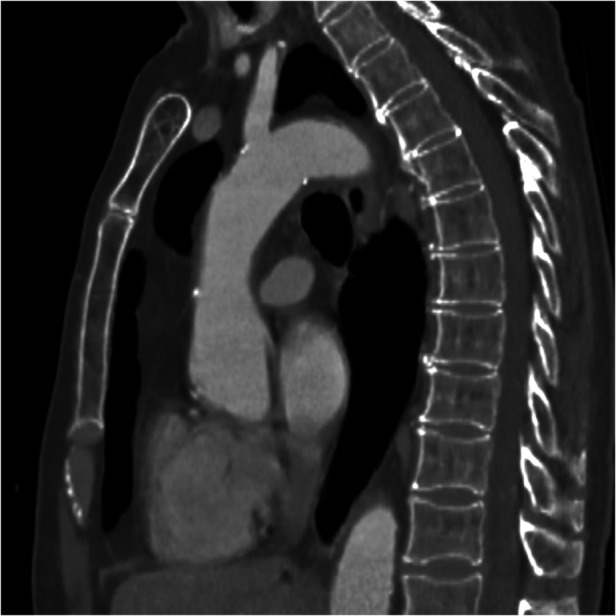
Sagittal CT-scan plane showing the resolution of the 8 mm thrombus in the ascending aorta.
